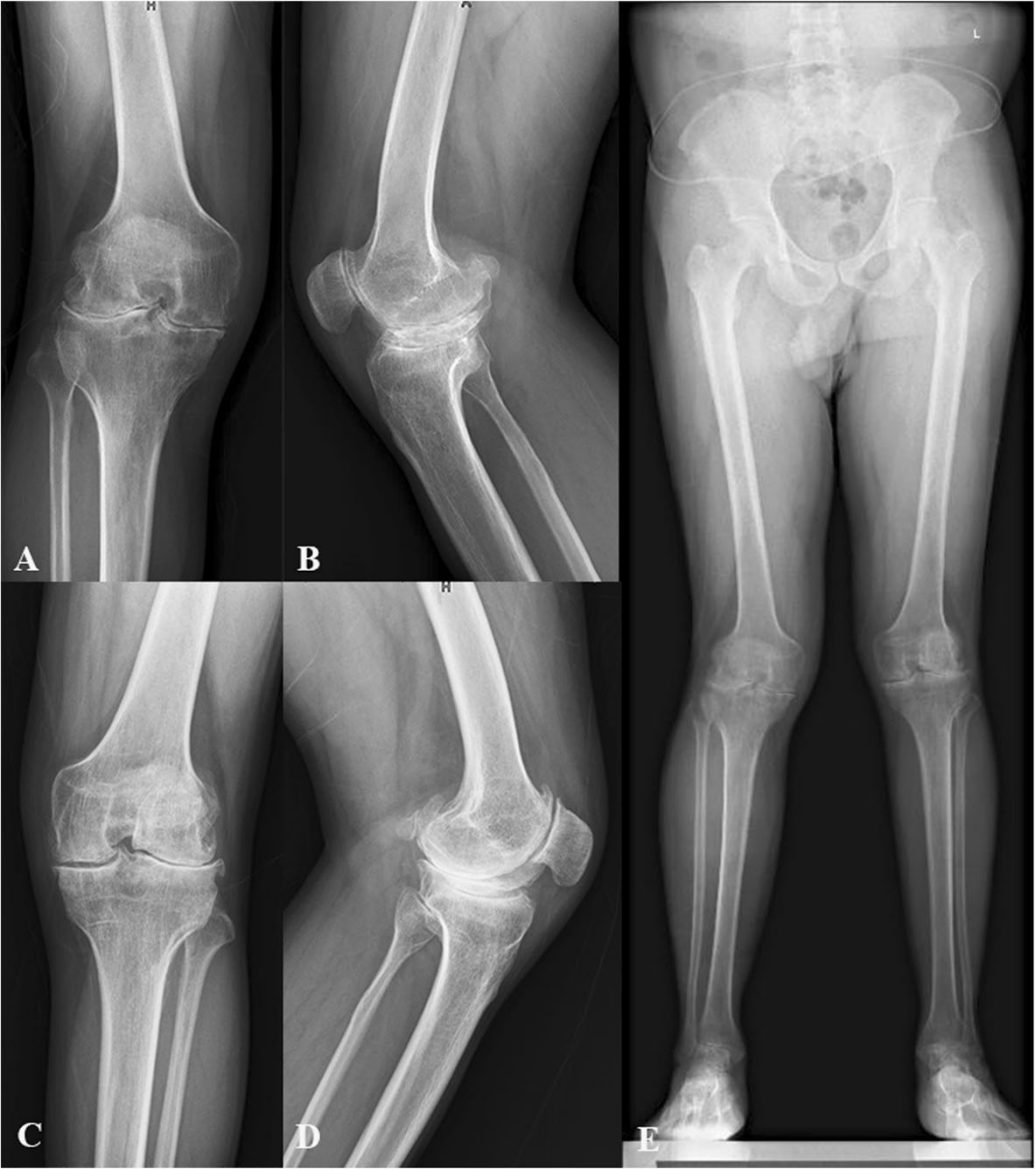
Preoperative X-ray of haemophilic arthropathy of a patient seeking for bilateral TKA. a Anteroposterior (AP) view of right knee; b Lateral view of right knee; c Anteroposterior (AP) view of left knee; d Lateral view of left knee; e Full-length standing AP view
